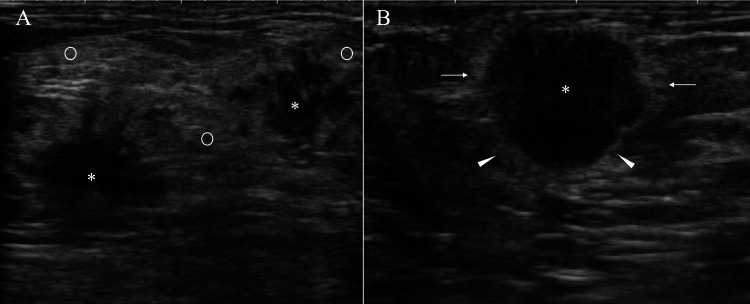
Ultrasound findings (A) Ultrasound showed two irregular masses (asterisks) with internal low echoes, indistinct margins, and widespread high echoes (open circles) around the masses. (B) Ultrasound of another breast cancer patient (the same case as Figure 1B) showed a low-echoic mass with halos not only at the anterior borders of the mass but also at the lateral (arrows) and posterior (arrowheads) borders.
